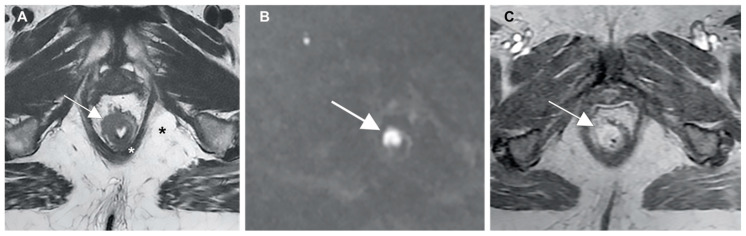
(A) Anal cancer captured using MR imaging, showing (white arrows) a higher signal intensity than the mucosal and submucosal layers; (B) restriction of proton diffusion at the DWI sequence; and (C) post-contrast enhancement after gadolinium injection.
